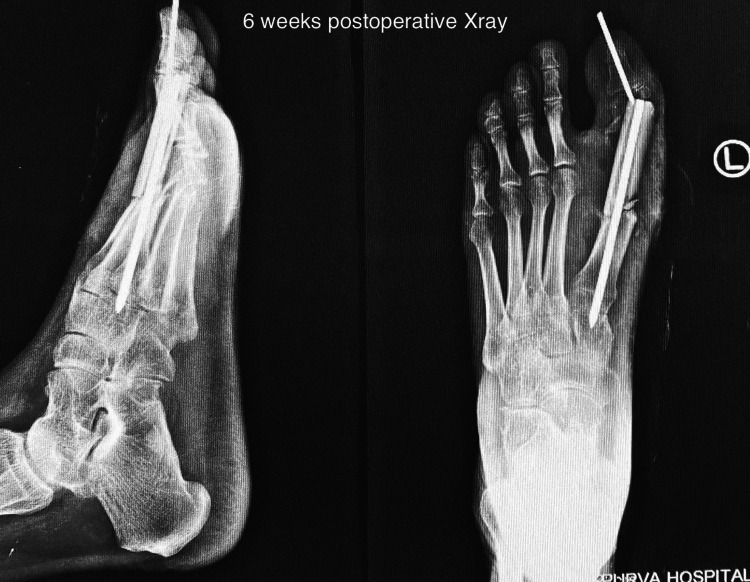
Six weeks postoperative X-ray showing early signs of union
